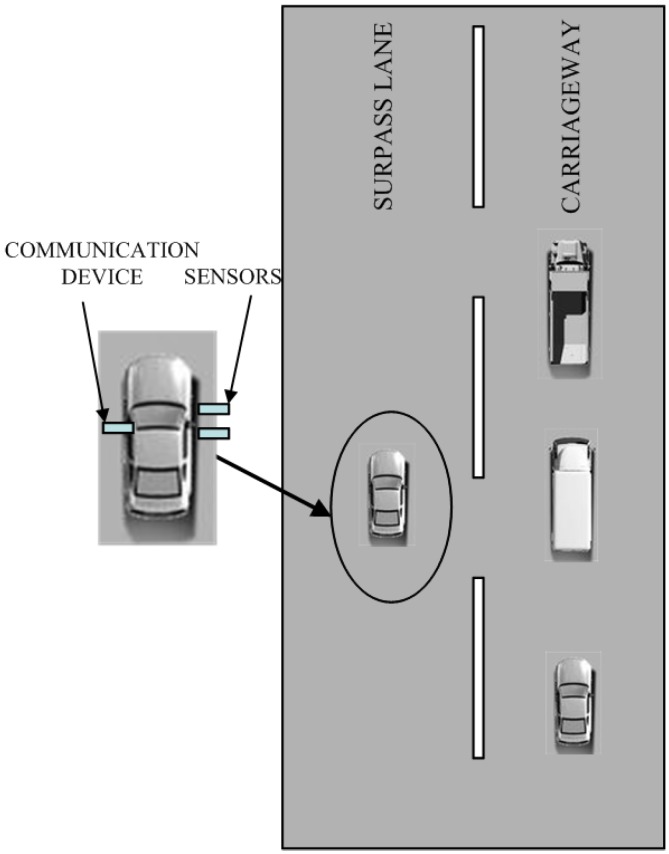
Ultrasonic sensor system for highway vehicle TRV identification.
